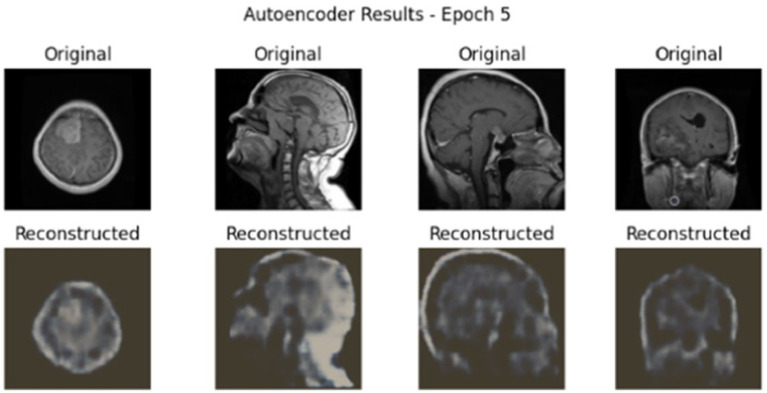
AE reconstruction results for MRI brain scans at epoch 5 on Dataset I.
